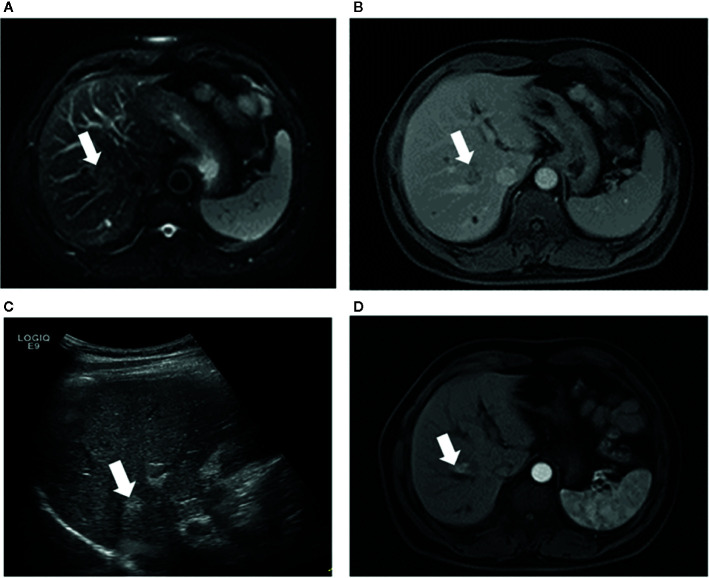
Images in a 53-year-old-man who underwent RFA for perihepatic CLM. (A) Axial MRI T2-weighted images shows a 2.6-cm lesion of high signal intensity (arrow) in segment VIII before RFA. The index tumor abuts the hepatic vein. (B) Axial enhanced MRI image shows that the lesion (arrow) washes out in equilibrium phase; (C) US image before RFA shows that the index tumor (arrow) is in contact with the hepatic vein. The patient underwent RFA, obtained technical effectiveness 1 month after RFA. (D) Axial enhanced MRI image showed local tumor progression around the ablation zone 6 month after RFA. CLM, colorectal liver metastases; US, ultrasound; RFA, radiofrequency ablation.
